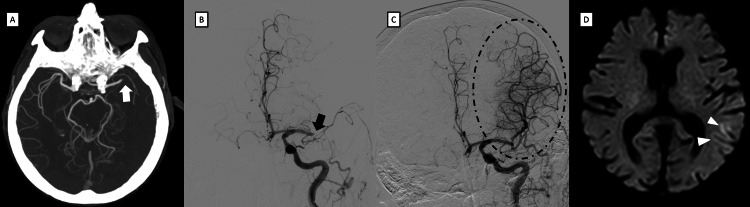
Images of our patient from admission to two days after MT. (A) Computed tomography angiography showing occlusion of the M1 segment of the left middle cerebral artery (white arrow). (B) Cerebral angiography showing distal occlusion of the M1 segment of the left middle cerebral artery (black arrow). (C) Complete recanalization was achieved with the first-pass effect using the combined technique (dot circle). (D) Magnetic resonance diffusion-weighted imaging two days later showed only slight infarcts in the left temporal lobe (white arrowheads). MT: mechanical thrombectomy.
